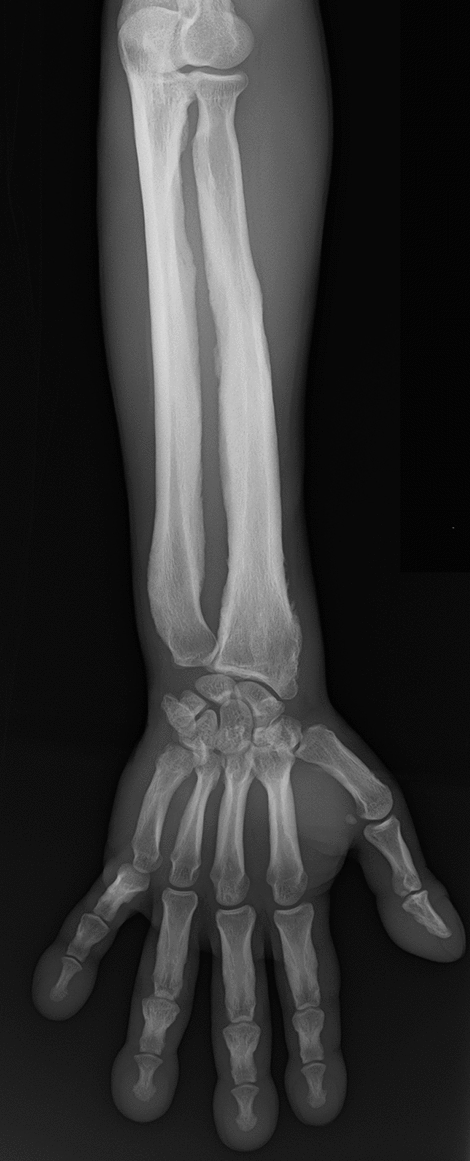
A 26-year-old male with pachydermoperiostosis. Radiograph shows irregular periosteal reaction of the short tubular bones and the long bones. Digital clubbing is also seen
